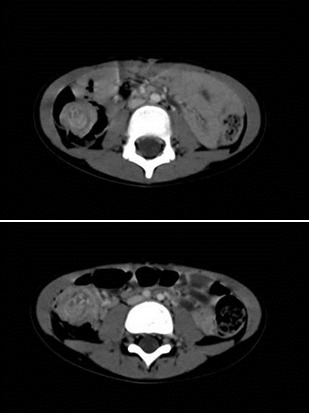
Aspects of CT scan at admission
